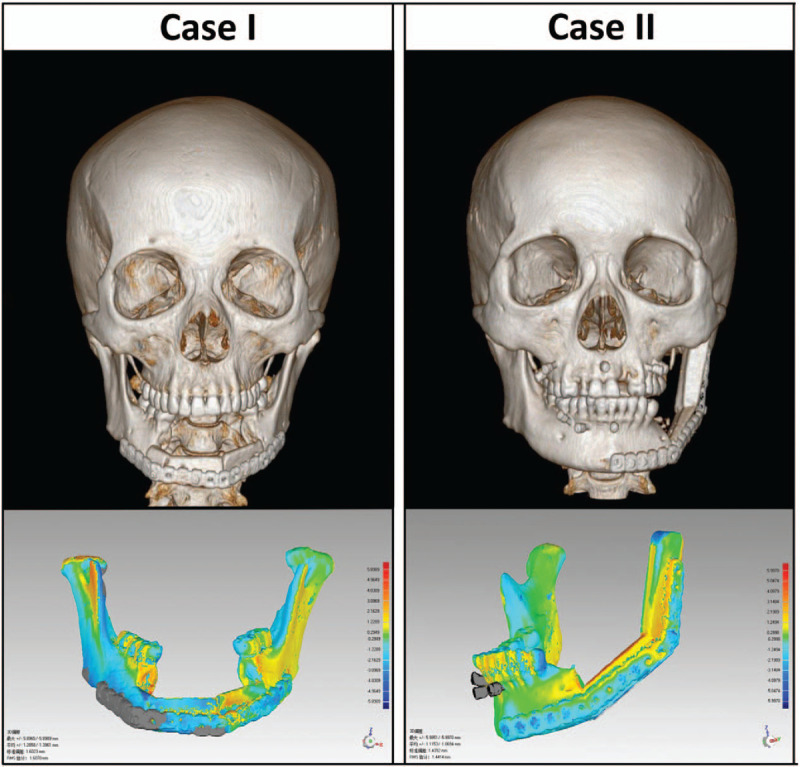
Postoperative CT and image fusion of the preoperative virtual design and postoperative 3D model. 3D = 3-dimensional; CT = computed tomography.
